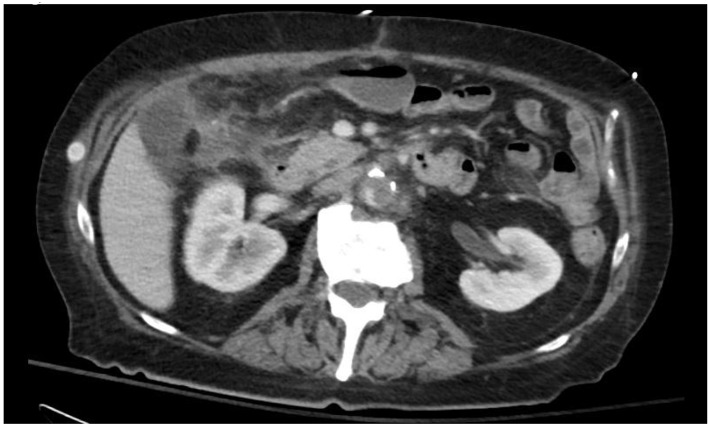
Coronal CT showing mycotic aneurysm of the infrarenal aorta with gas bubbles.
